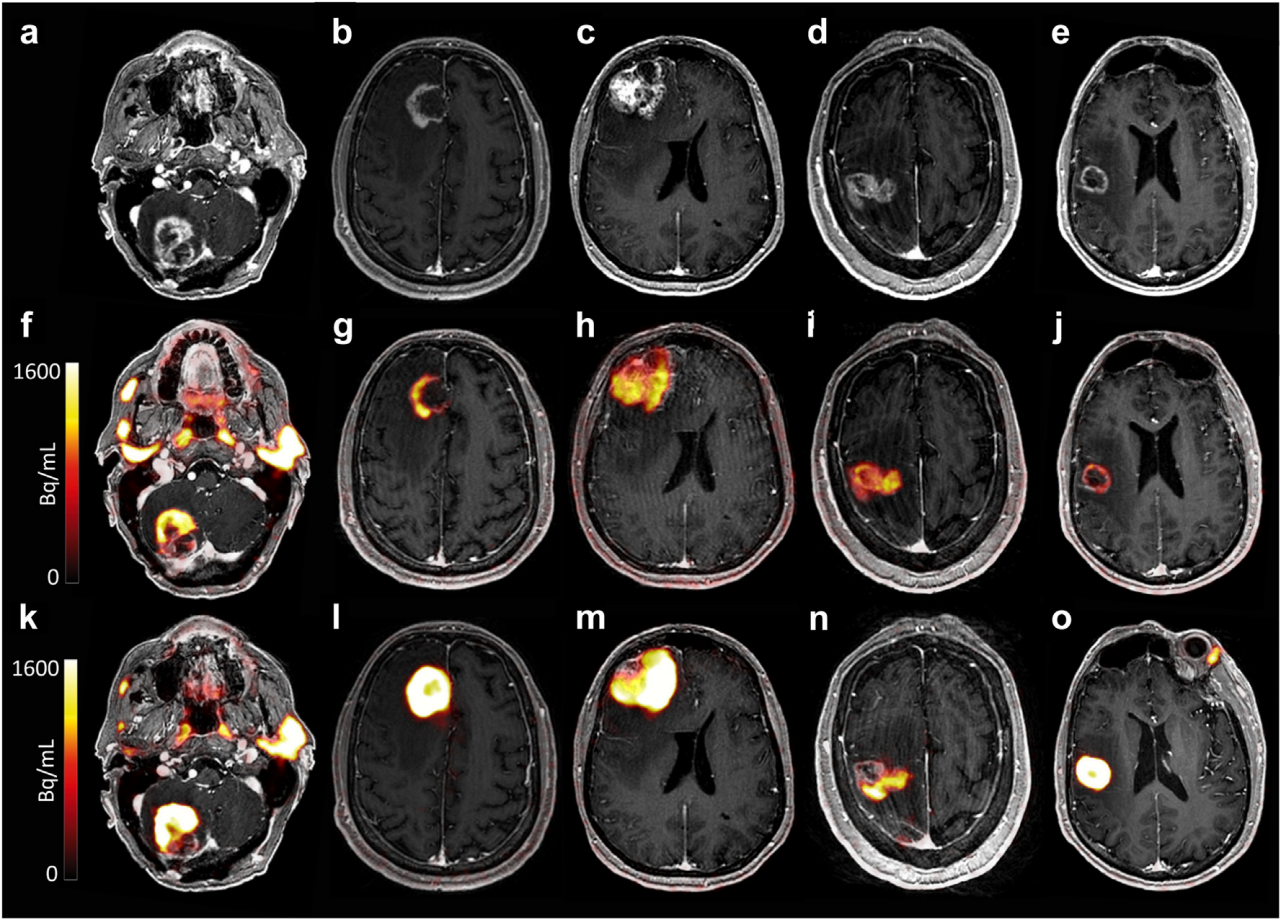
Uniformly scaled representative images of each patient with BM (from left to right, no. 6–10) including a post-contrast T1w MR image (a–e), and fused [^68^Ga]Ga-PSMA-11 PET with post-contrast T1w MR images after IV (f–j) and ssIA administration (k–o). Abbreviations: BM: brain metastasis; IV: intravenous; PSMA: prostate-specific membrane antigen; ssIA: super-selective intra-arterial.
